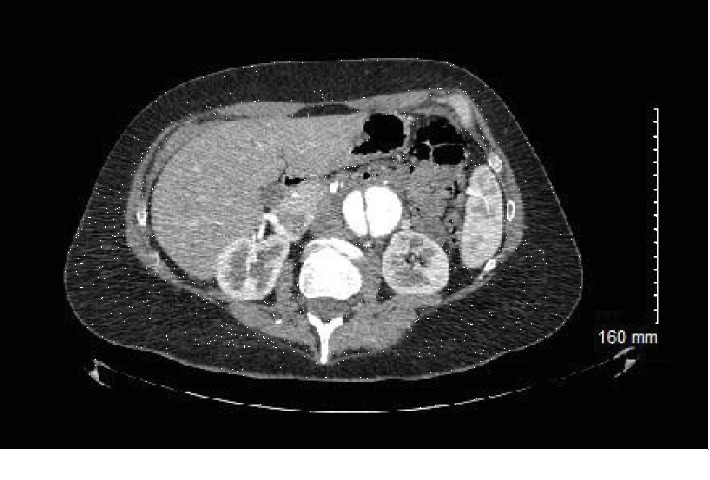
CT scan of the abdomen/pelvis with IV contrast demonstrating dissection of the abdominal aorta.
